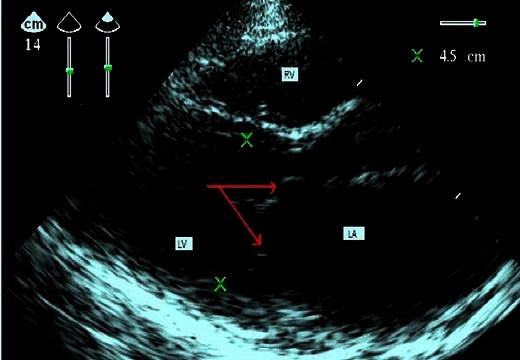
Parasternal long-axis 2D echocardiographic image in diastole showing the incomplete opening of the mitral valve. (LA: left Atrium, LV: left ventricle, RV: right ventricle, arrows: thickening of the large and small mitral valve)
